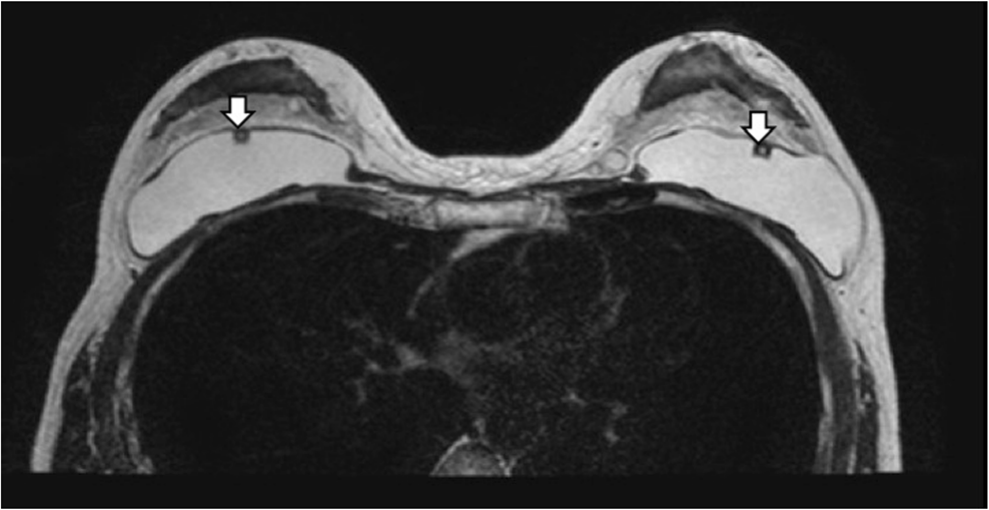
Saline-filled implants. High signal intensity identical to water is seen in saline-filled implants on T2-weighted sequence. Note the valves (arrows) at the anterior aspect of the shells, which are characteristic of saline-filled implants, but not silicone gel-filled implants
